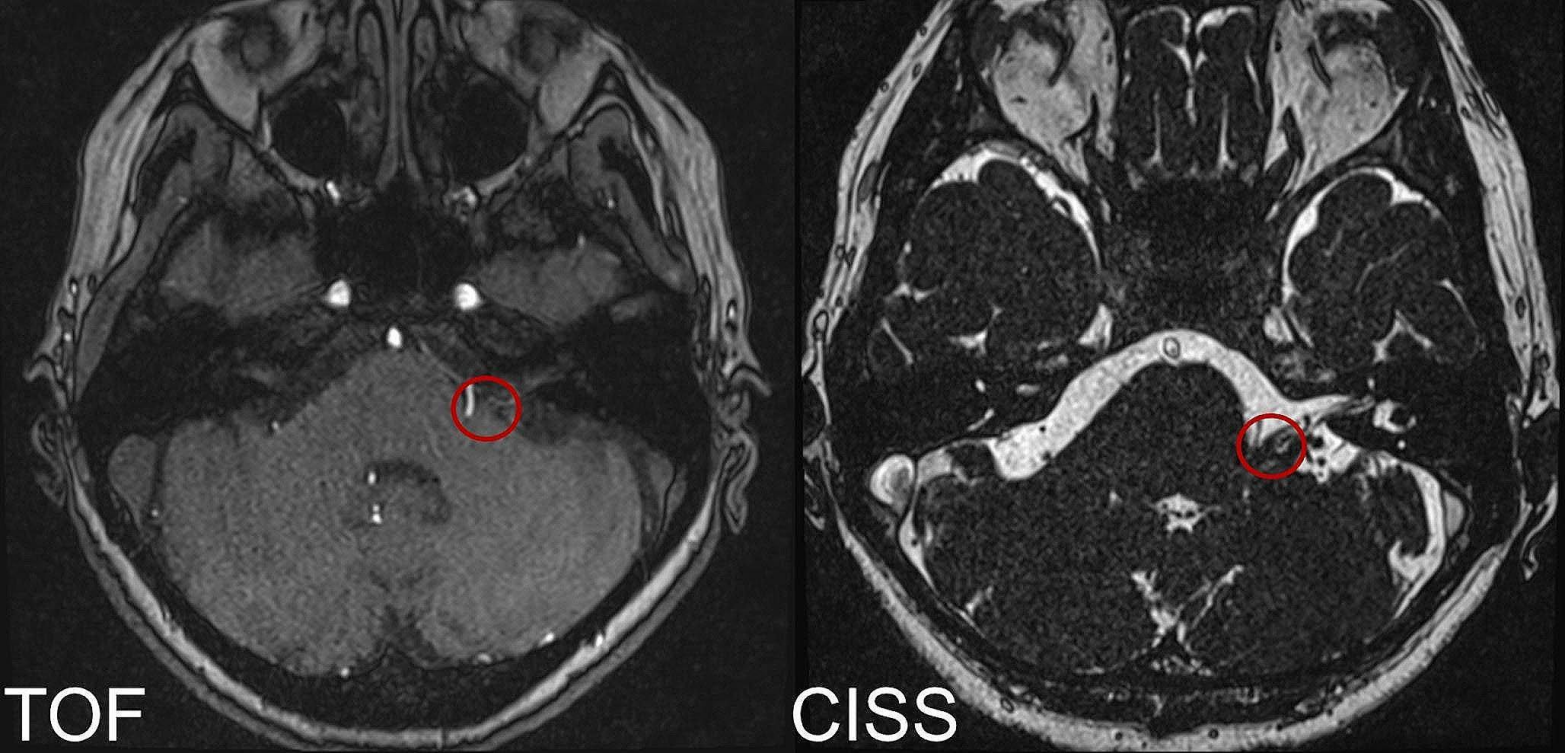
Preoperative axial TOF and CISS MRI sequences demonstrate a potential conflict (red circle) between the PICA and the left facial nerve at its root exit zone, near to the brainstem´s surface
